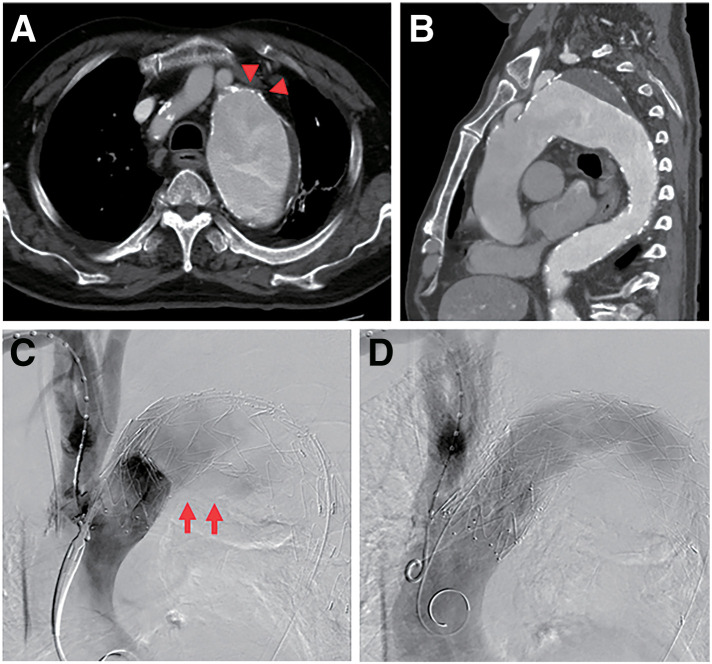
Preoperative CECT and intraoperative aortography during 2-debranching TEVAR. (A) Axial and (B) sagittal CECT showing a 67-mm distal aortic arch aneurysm with calcification in the proximal landing zone (arrowheads). (C, D) Intraoperative aortography during 2-debranching TEVAR. (C) Type Ia endoleak after initial proximal stent graft deployment (arrows). (D) Resolution of the endoleak after additional relining. No evidence of brachiocephalic artery dissection at this stage. CECT: contrast-enhanced computed tomography; TEVAR: thoracic endovascular aortic repair
